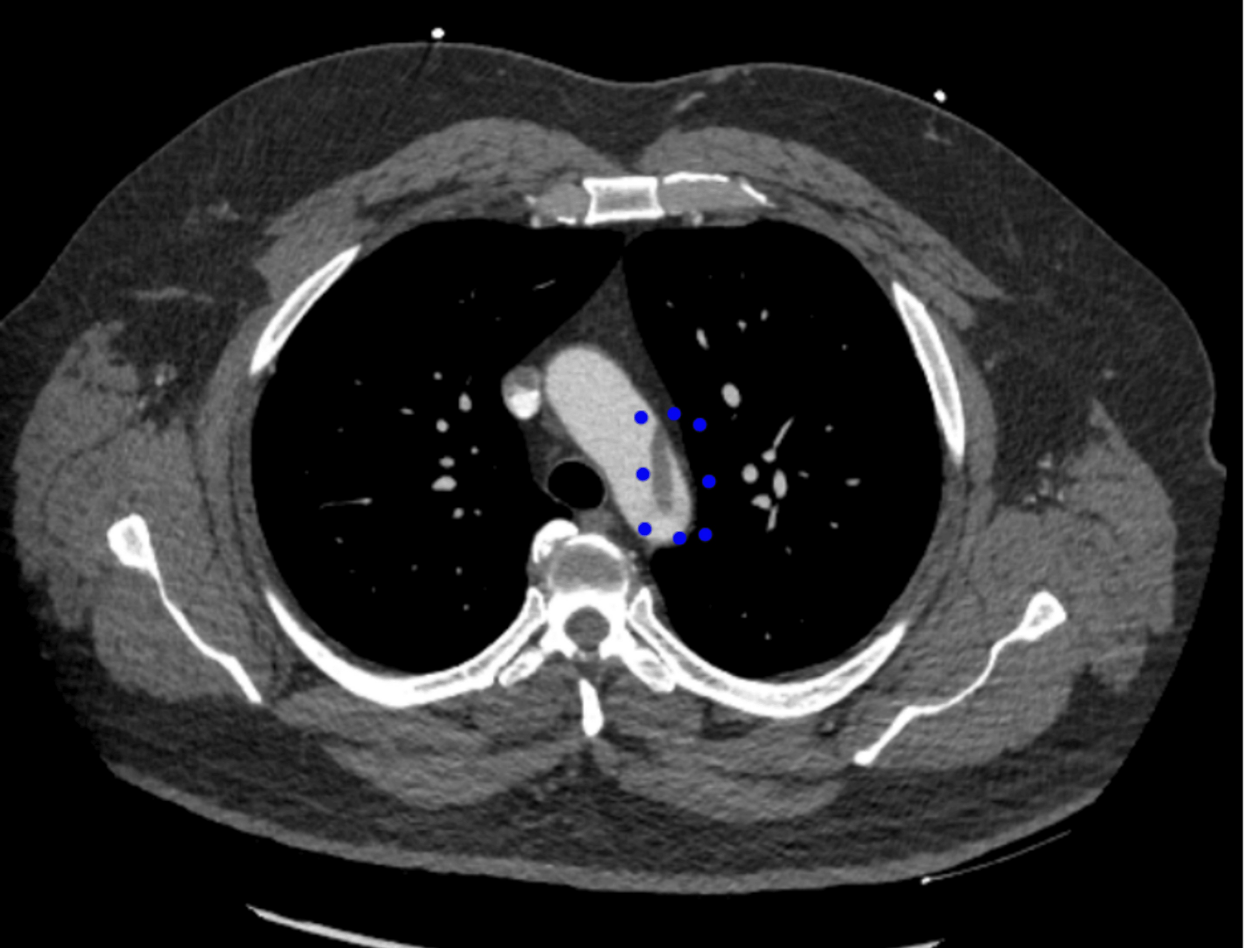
CT scan of the chest demonstrating the aortic mural thrombus This image shows the CT scan of the chest with IV contrast that was taken highlight the mural thrombus noted in the arch of the aorta with blue dots.
